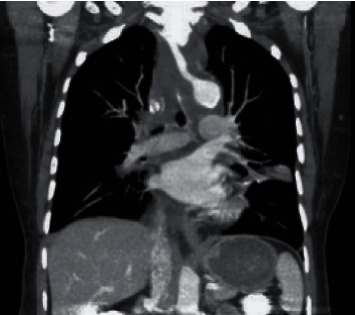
CT scan demonstrating ill-defined fluid collection in the superior mediastinum (arrow).
